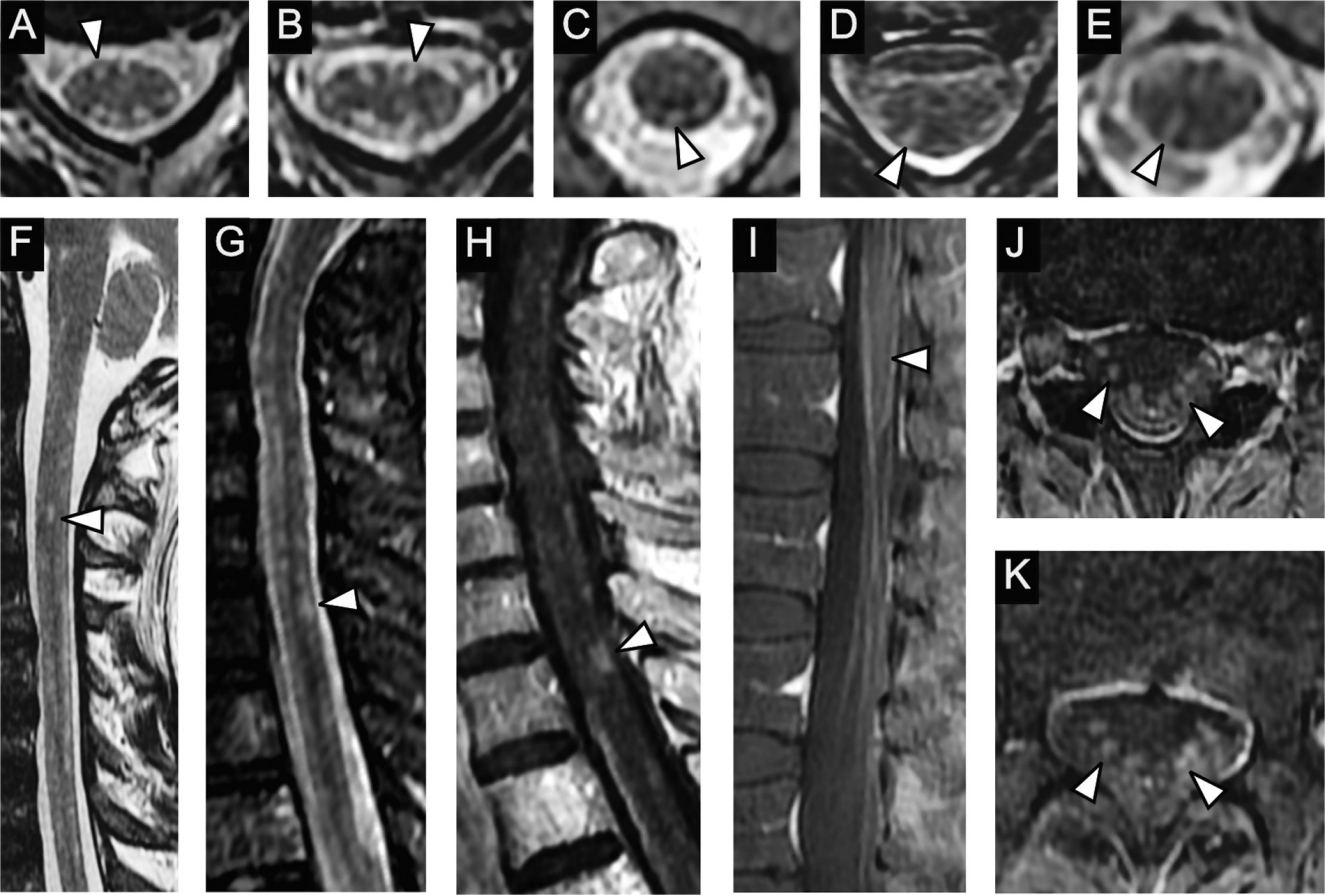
Spinal cord magnetic MRI features of patients with CHIKV-associated encephalomyelitis. (A, B) patient 7 - Axial FSE T2WI images demonstrating small dotted lesions (arrowheads) with high signal in the periphery of the spinal cord (the “
